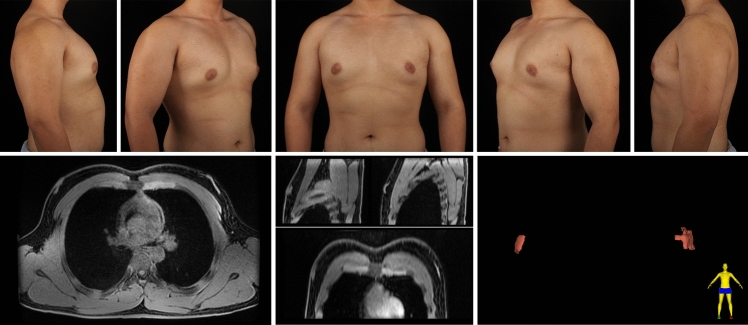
Type 2A gynecomastia. Preoperative chest appearance (upper) and breast MR images (lower) of an 18-year-old man with type 2A gynecomastia
